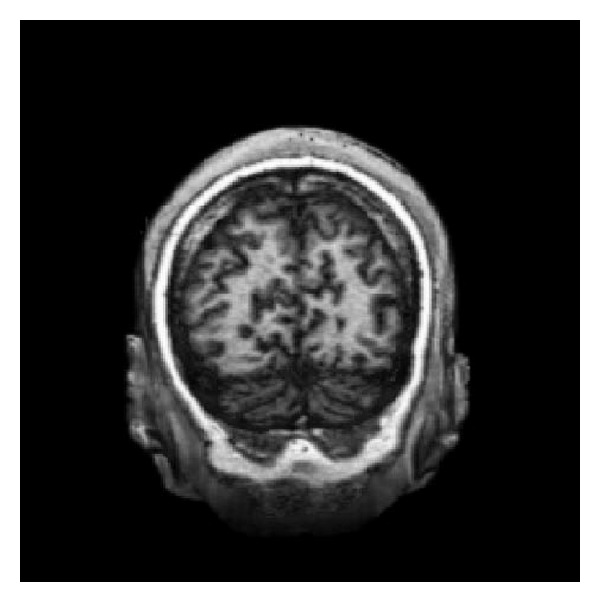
An example of MR image: 3D view from behind with part of hindbrain clipped.
